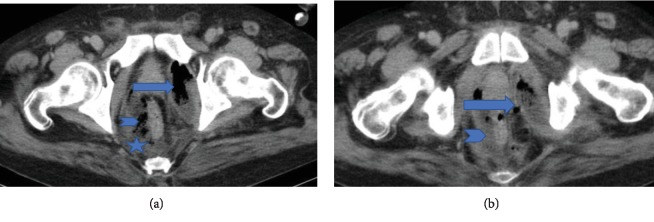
(a) Axial CT scan of the abdomen and pelvis through the hip joints showing abscess (gas and fluid) in the left obturator internus muscle (arrow). Gas (arrowhead) and fat stranding (asterisk) are also seen in perirectal space. (b) Axial CT scan of the abdomen and pelvis at level below the hip joints showing site of the left OIM abscess rupture (arrow). In addition, inflammatory fluid (arrowhead) is seen in perirectal space.
